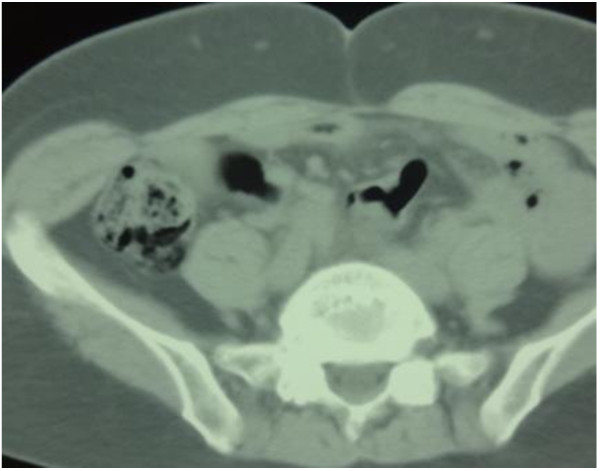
Abdominal computed tomography scan examination showing extensive appearance of intramural gas in the colon.
